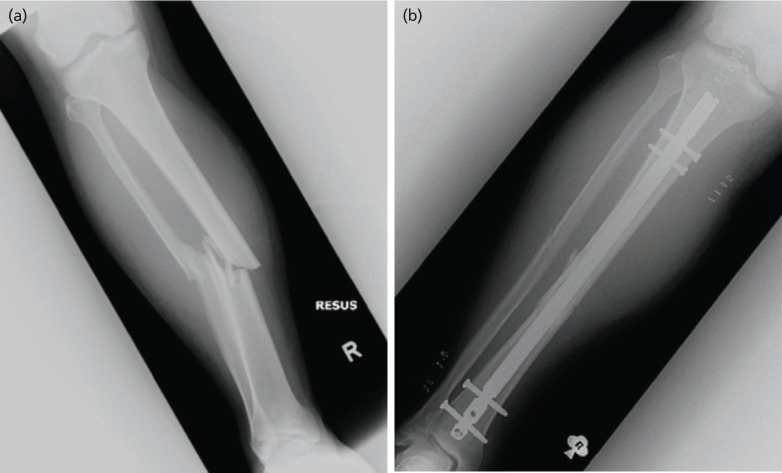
Radiograph images of Case 3. (a) Initial injury on arrival. (b) Post-operative follow-up.
